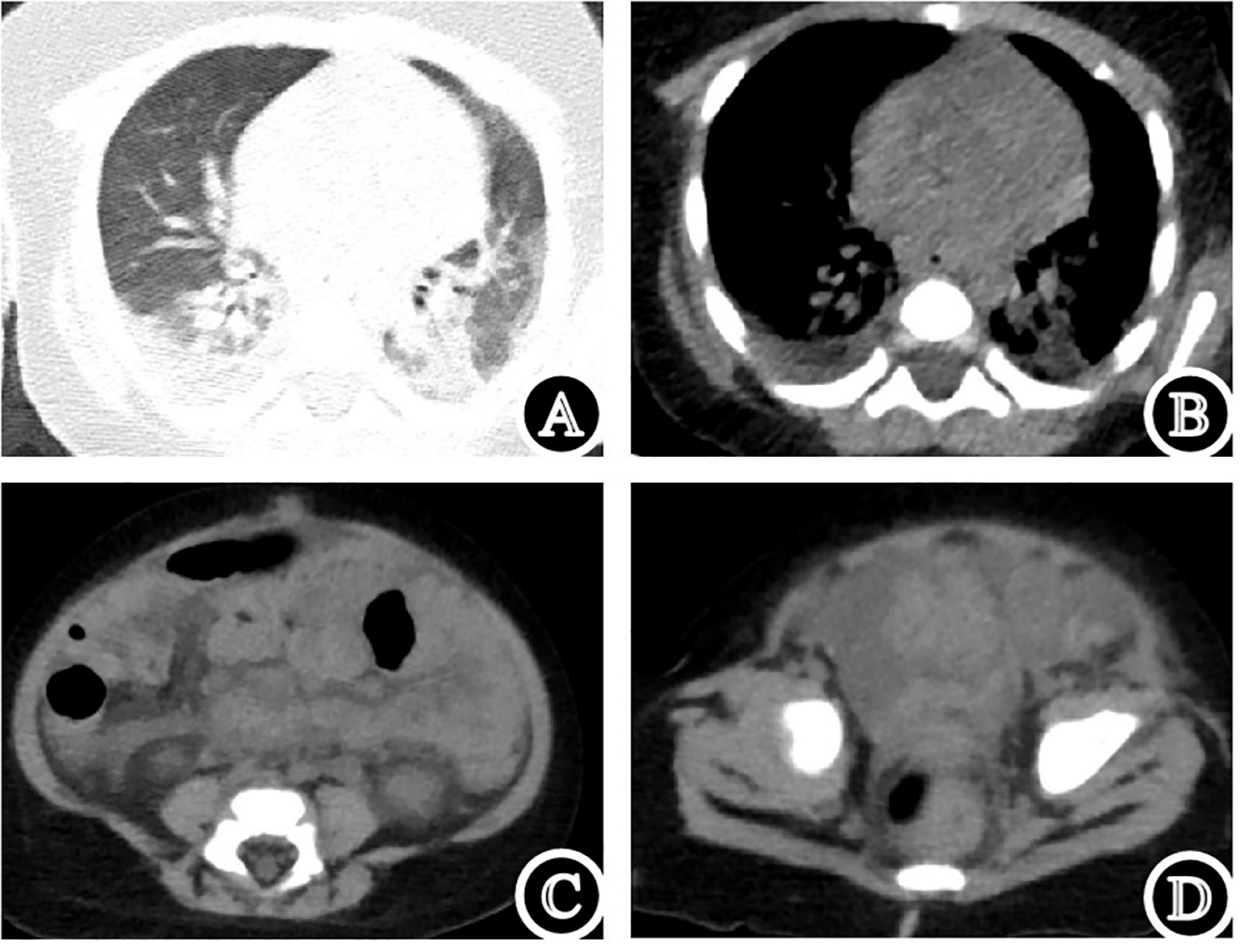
Clinical images of the patient. (A, B) Chest computed tomography (CT). (C, D) Abdominal and pelvic CT.
